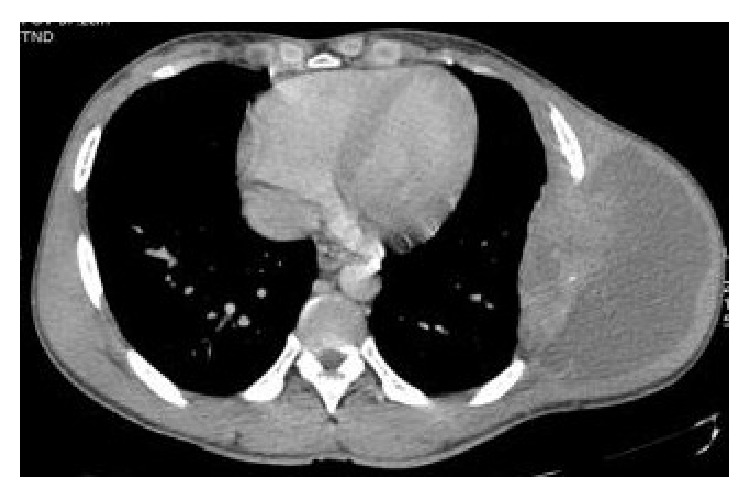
Axial Computed Tomography image at lesion level in mediastinal window showing well loculated hypodense collection in the left chest wall with peripheral enhancement.
